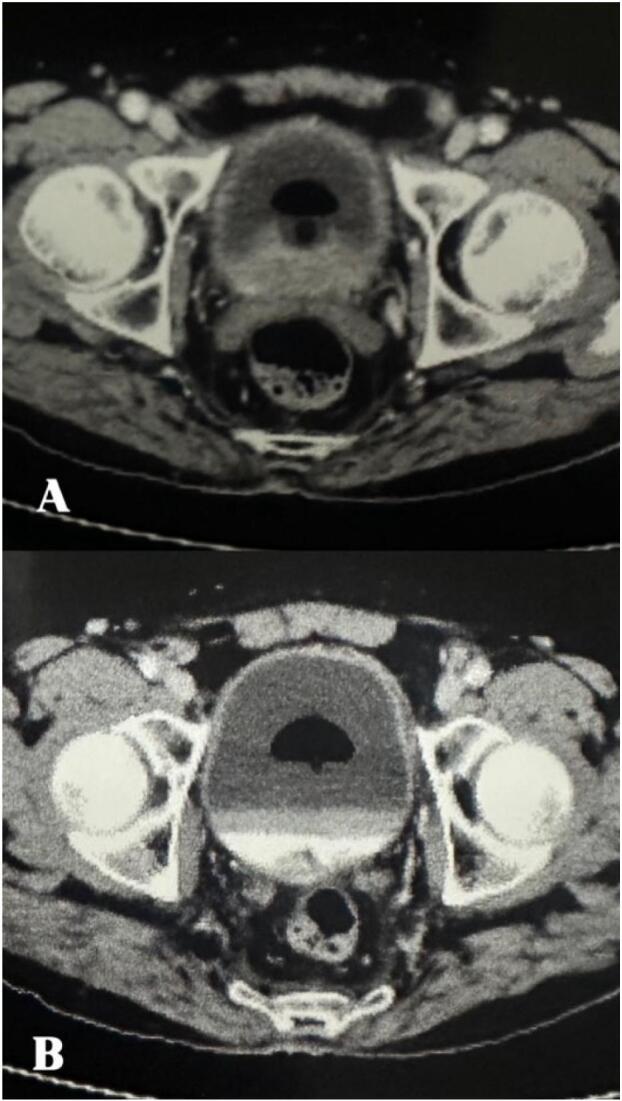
(A,B)- Contrast enhanced CT scan of bladder showing bubl of foley above the clot and debris.
